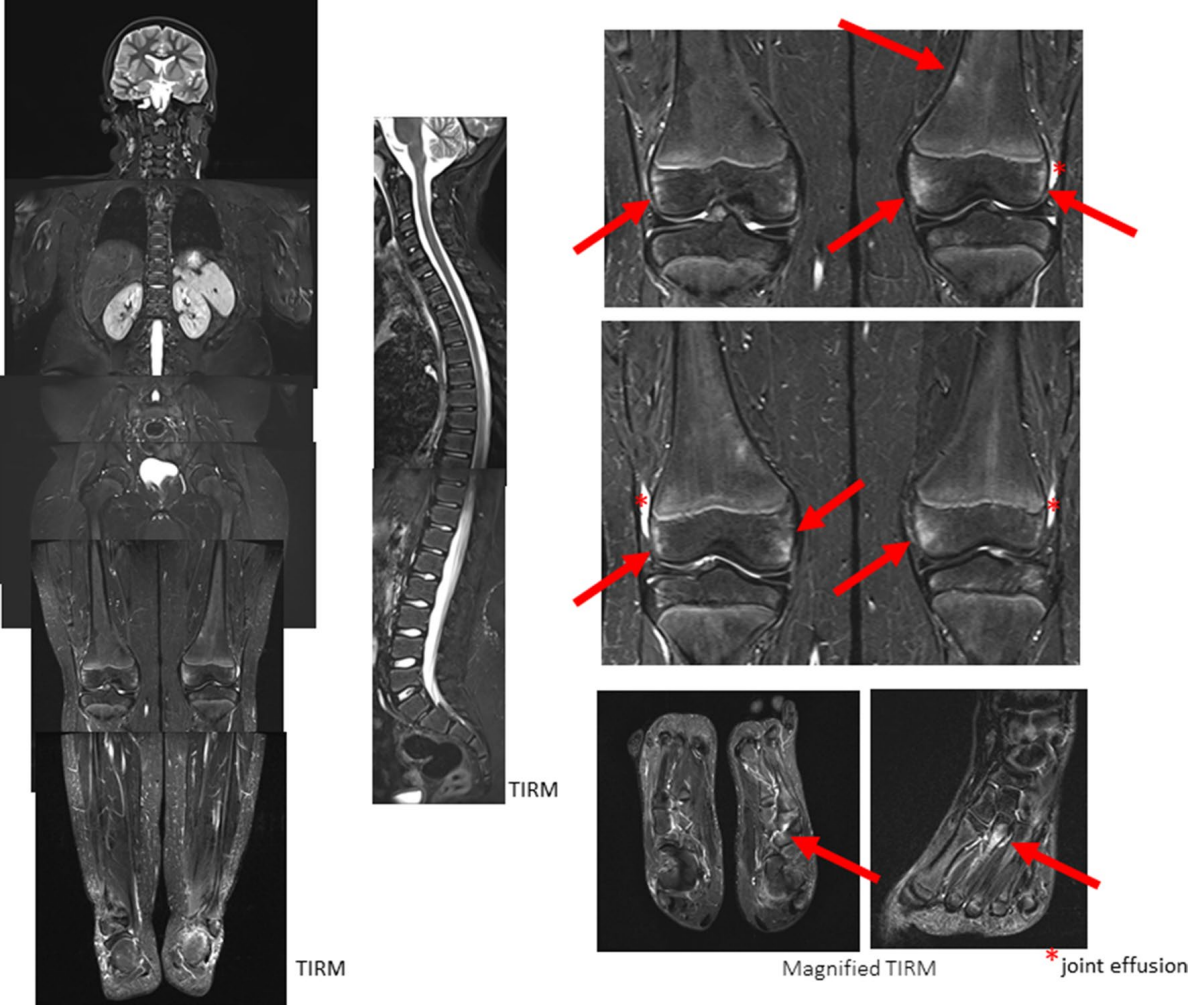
An 11-year-old boy also without previous history of MSK pathologies and pain at multiple body regions (lower extremities, especially knees and feet). MRI shows bone marrow edema—more subtle than in Fig. 1, affecting the long bones (with focus on epiphyseal and metaphyseal areas) and MT
